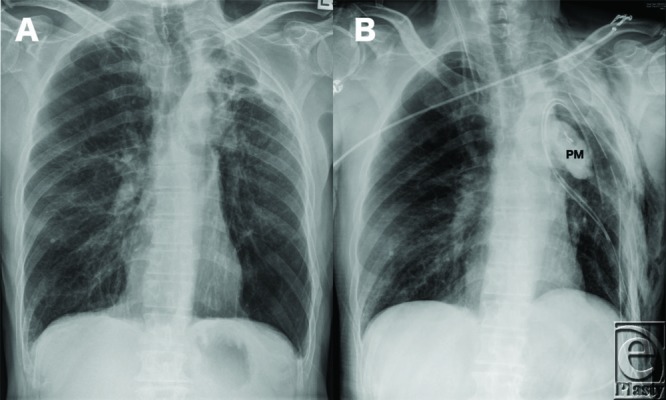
(*a, b*) Preoperative and postoperative plain chest x-rays. The pectoralis major (PM) flap obliterating the intra-thoracic dead space resulting from the upper lobectomy.
